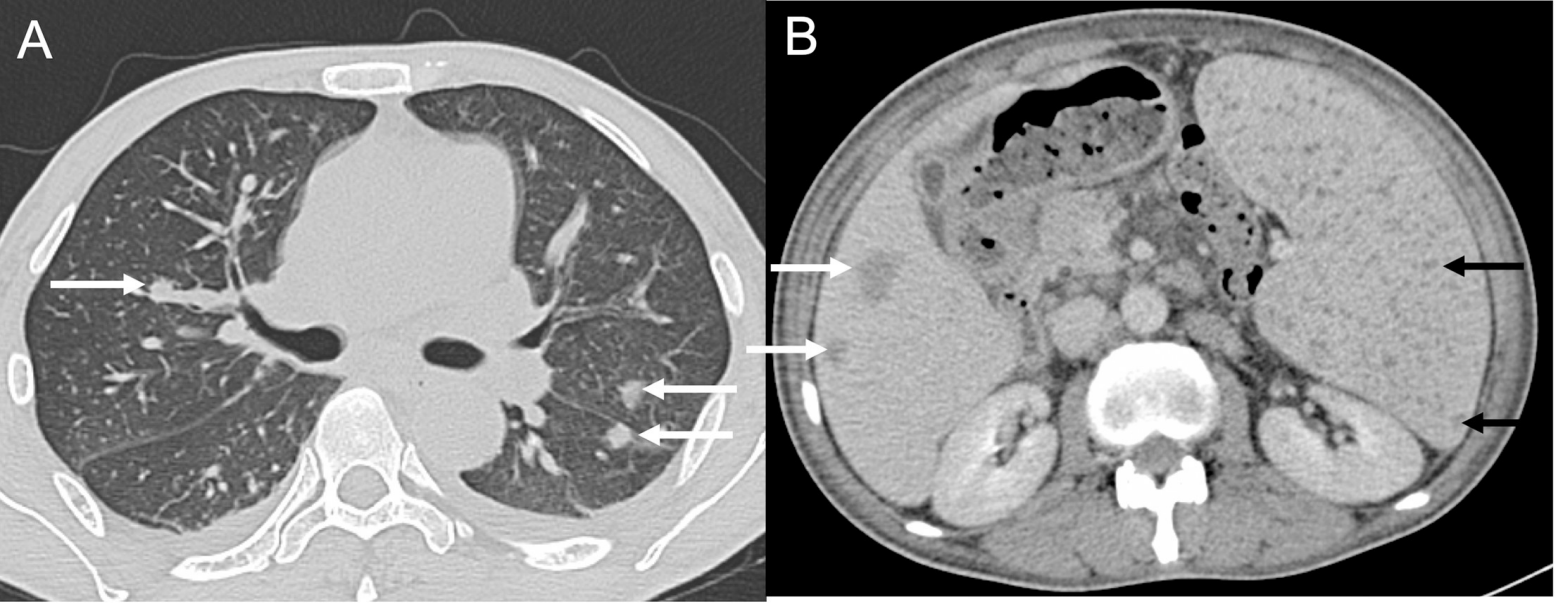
Clinical images from the patient. (A). An image of abdominal CT. Lesions of the liver are shown by white arrows, and nodes of spleen are shown by black arrows. (B). Spleen that was removed from the patient. Nodes in yellow are pointed out by arrows. CT, computed tomography.
